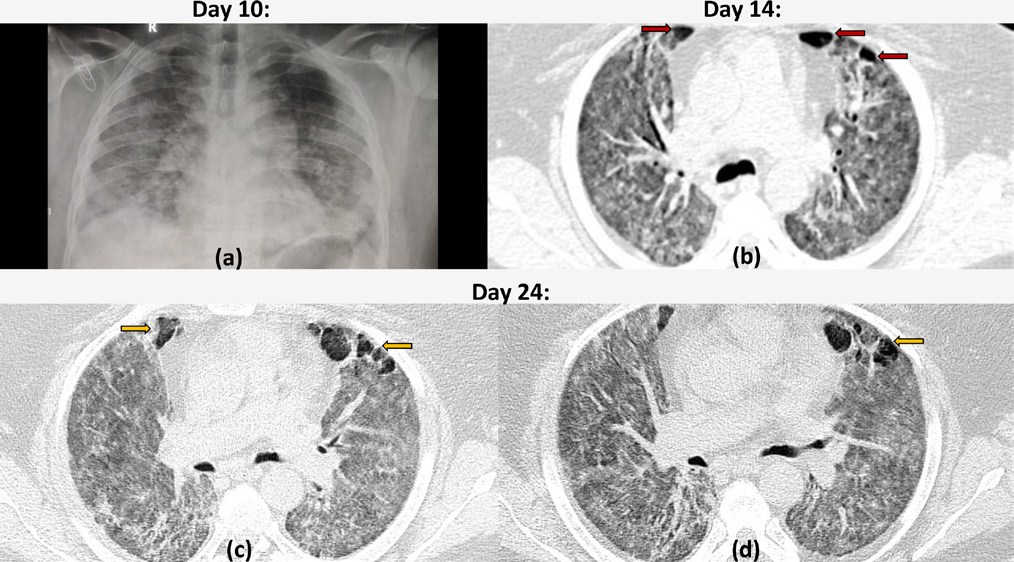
Multiple cystic air spaces in severe COVID19 pneumonia: 53-year-old male with COVID19 infection and severe shortness of breath and low oxygen saturation. Chest radiograph (a) on Day 10, shows multifocal fluffy air space opacities in the bilateral lung fields with an apicobasal gradient. Chest HRCT (axial section a) done on Day 14 of illness shows bilateral diffuse ground glass opacities with interlobular septal thickening. A few cystic air spaces (with regular inner walls) are seen anteriorly in para-septal location (red arrows) (within the middle lobe and lingular segment of left upper lobe) bilaterally. HRCT on Day 24 (c, d) revealed an increase both in number and size of the cystic air spaces (yellow arrows) while the parenchymal changes have become sharper and more organized, although still severe.
